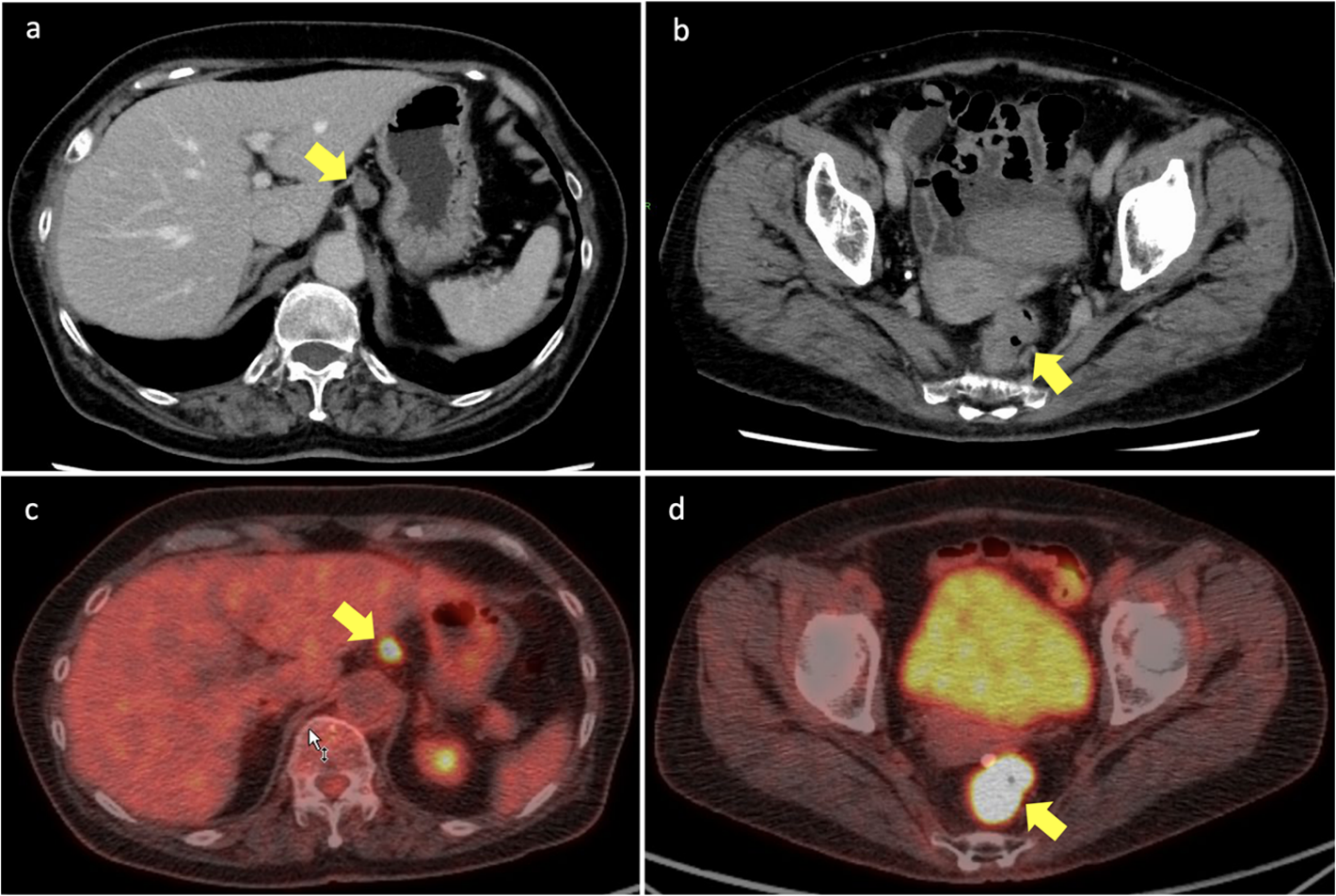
Computed tomography (CT) and fluorodeoxyglucose positron emission tomography (FDG-PET). a Enhanced upper abdominal CT showing three lymph node swellings in the lesser curvature area of the stomach (arrow). b Enhanced lower abdominal CT revealing rectal cancer invading the serosa (arrow). c Upper abdominal FDG-PET CT showing FDG accumulation in the lesser curvature lymph nodes of the stomach (arrow). d Lower abdominal FDG-PET CT scan revealing FDG accumulation in the main tumor of the rectum (arrow)
